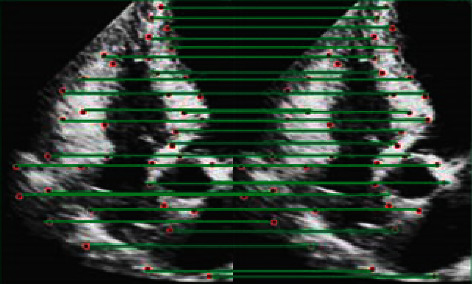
Matching results of cardiac ultrasound images in frames 1-2.
